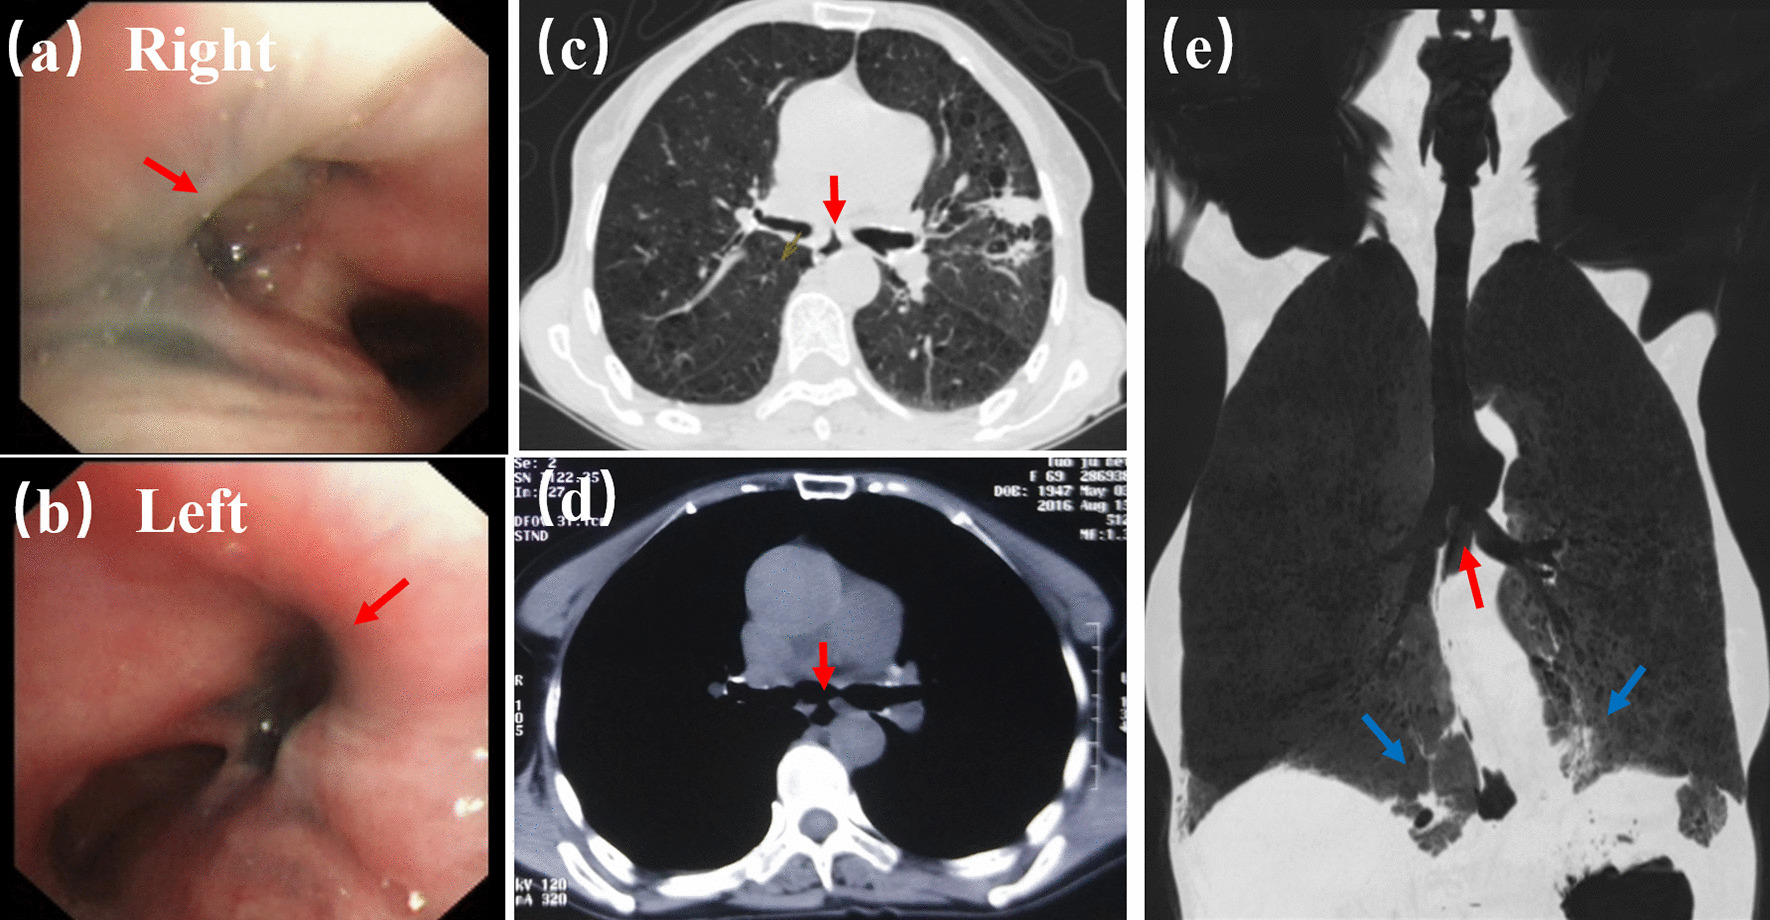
Preoperative imaging examination (red arrows indicate opening fistula, while blue ones indicate lung pneumonia). a, b Bronchofiberscopy showing a double-opening fistula in the posterior-interior wall of the trachea. Chest CT results demonstrating a three-way tracheoesophageal fistula (c, d) and pneumonia in the lower lung field (e)
